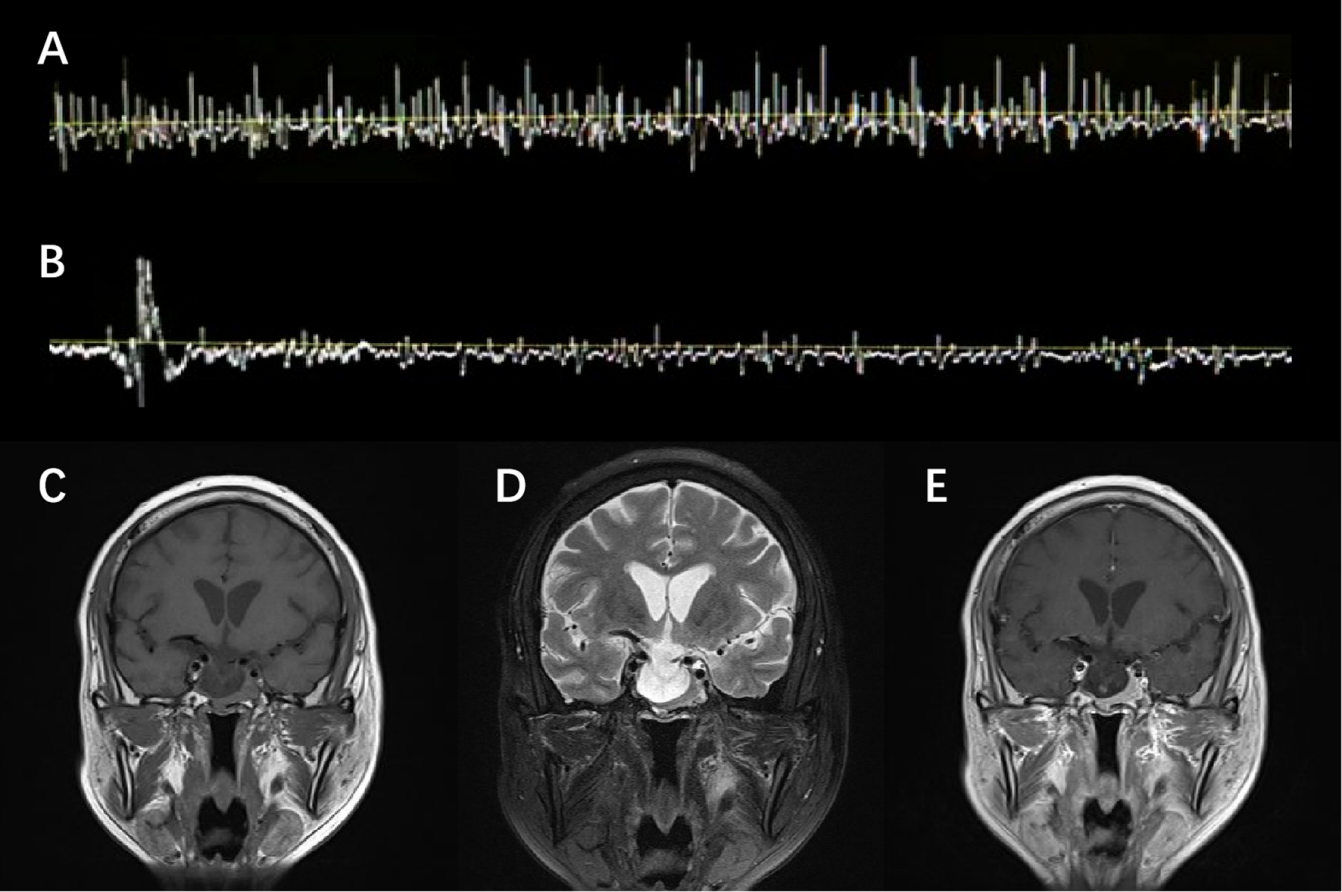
EMG and MRI presentation. EMG observed spontaneous but transient motor-unit potential firing of the right rectus abdominis at the resting state (A), which self-relieved in 4 min without diazepam (B). T1-weighted (C), T2-weighted (D), and Gd-enhanced T1-weighted (E) MRI scan of the brain showed a heterogeneously enhanced pituitary and empty sella.
